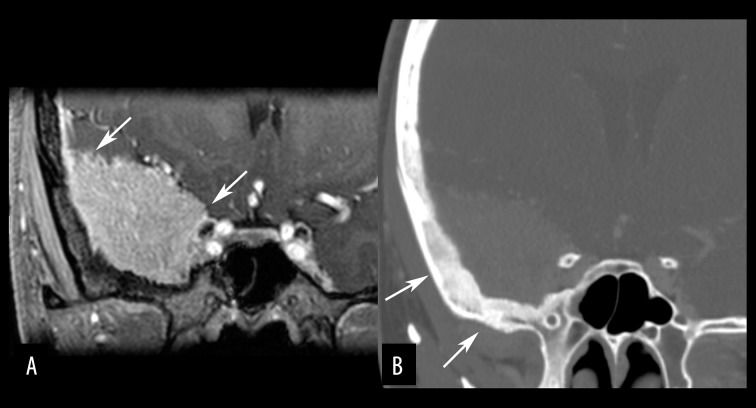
Skull base meningioma in a 29-year-old woman. (A) Coronal-reformatted, post contrast, T1-weighted image shows a meningioma with typical dural tail sign (arrows). (B) Coronal-reformatted, bone window CT image demonstrates hyperostosis of the underlying bones (arrows).
